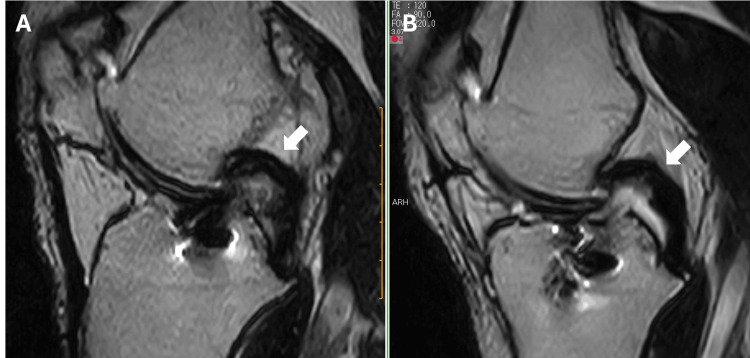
MRI showed that the front scar tissue of PCL was removed and the PCL buckling (white arrow) was reduced after arthroscopic surgery: (A) before arthroscopic surgery and (B) one year after arthroscopic surgery. MRI: magnetic resonance imaging; PCL: posterior cruciate ligament
